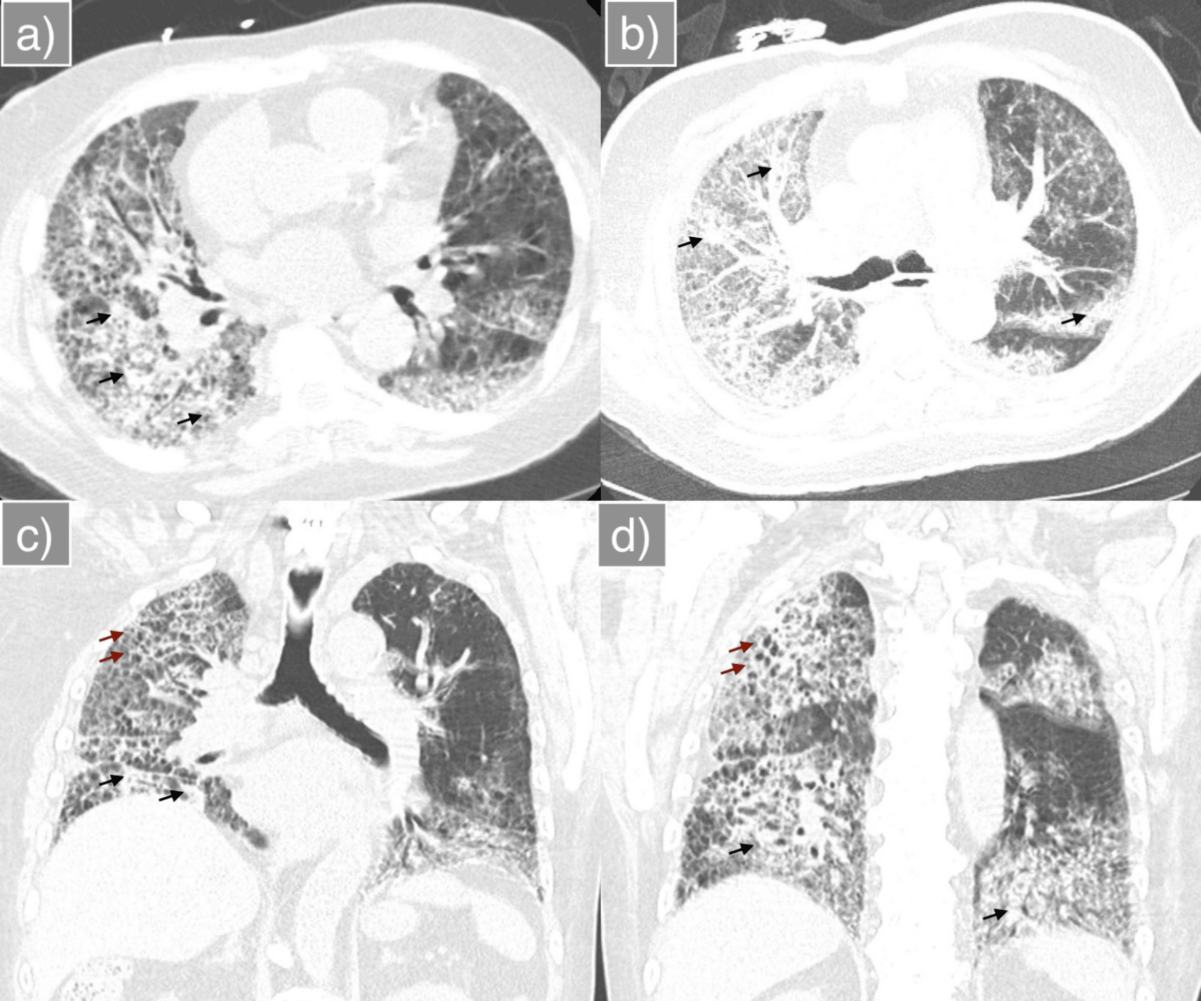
High-resolution CT of the chest demonstrating advanced fibrotic interstitial lung disease (a) Axial section at the mid-lower thorax (atrial level) showing advanced fibrotic changes with diffuse opacities (black arrow) and interstitial thickening, more pronounced on the right. (b) Axial section at the mid-thorax demonstrating similar findings with right-sided predominance. (c) Coronal section at the level of the tracheal bifurcation showing diffuse fibrotic changes, opacities (black arrow), ground-glass opacities, and honeycombing (brown arrow), more prominent on the right. (d) Coronal section anterior to the trachea demonstrating similar advanced fibrotic changes, opacities (black arrow), and honeycombing (brown arrow), predominantly on the right.
